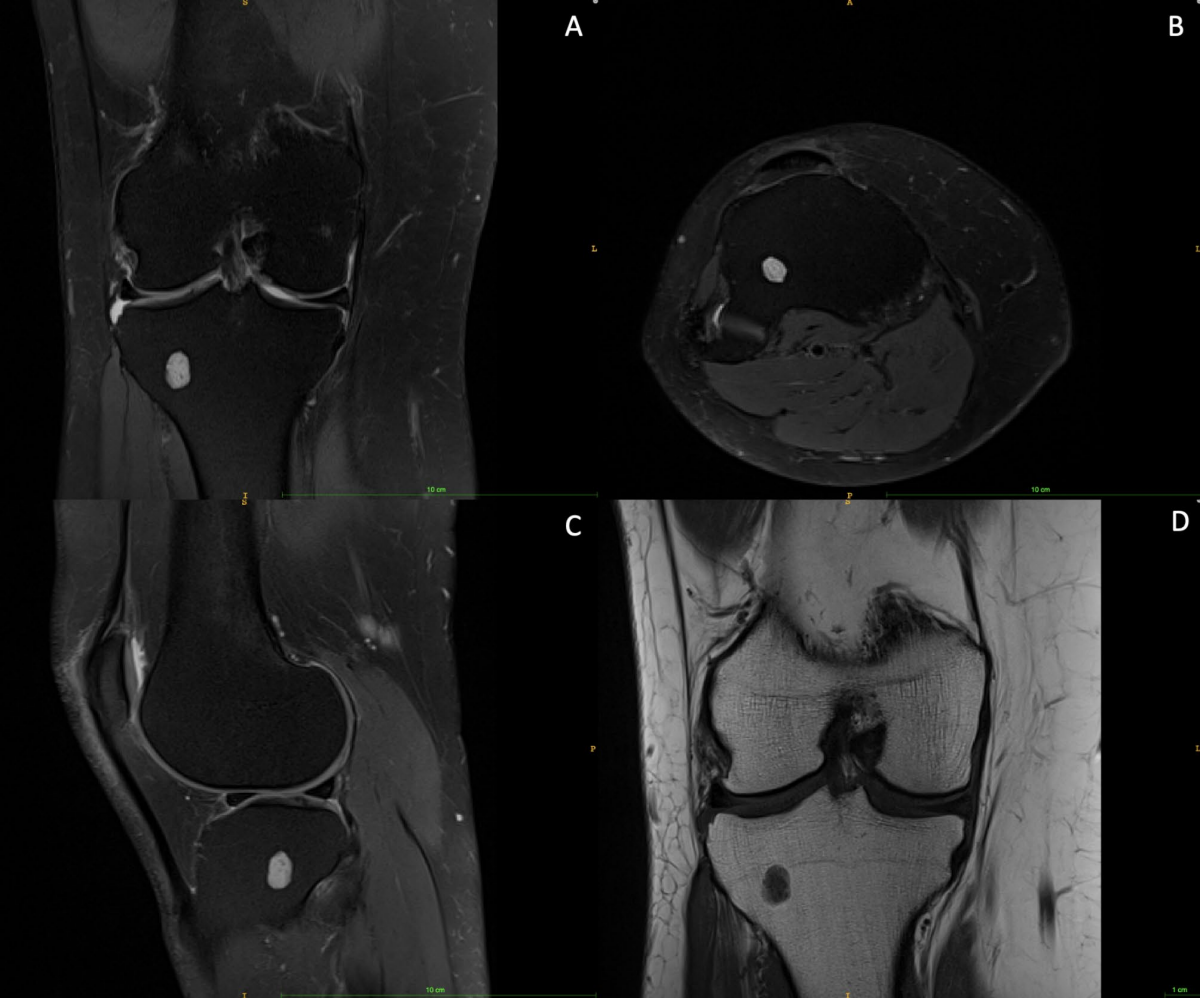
EC of the right tibia: (A) proton density, fat suppression, coronal, turbo spin echo; (B) proton density, fat suppression, transversal, turbo spin echo; (C) proton density, fat suppression, sagittal, turbo spin echo; (D) t1, coronal, turbo spin echo (year of MRI: 2016)
